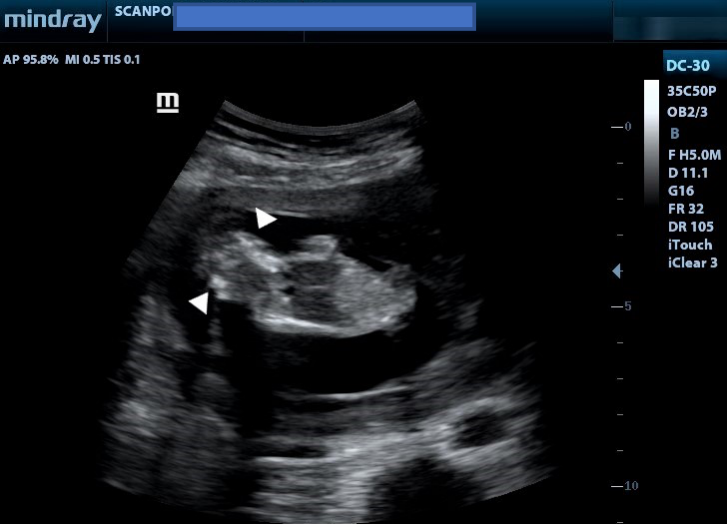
Coronal Ultrasound image showing a foetus at 13 weeks with no calvaria. White arrowheads show the brain tissue without bony covering. The amniotic fluid index (AFI) was 17 cm
